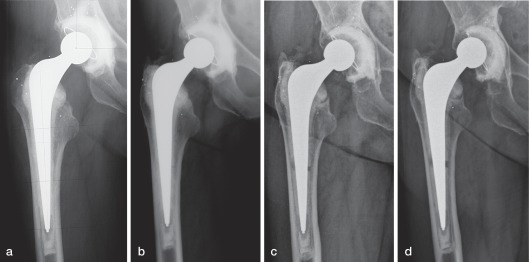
Patient 3 with a complete circumferential cement mantle fracture. a) 6 weeks post-operative radiograph: No abnormalities were seen. b) 2 year follow-up radiograph: A cement mantle fracture in Gruen zone IV is noted. c) 5 year follow-up radiograph: A complete circumferential cement mantle fracture is present. d) 10 year follow-up radiograph: Increased separation of the cement mantle. Between the 2 and 3 year RSA examination, a sudden and substantial increase in subsidence and retroversion of the stem was measured. At 12 year follow-up, subsidence was 9.7 mm and retroversion 11°.
